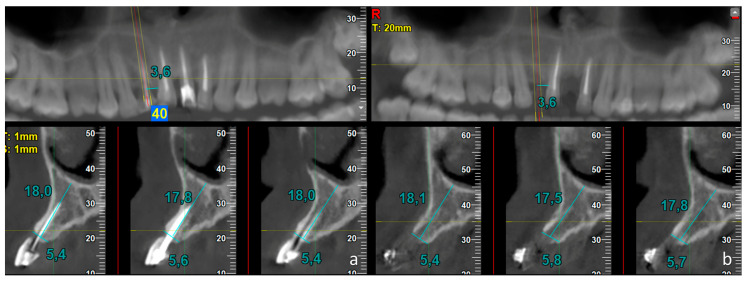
Test site. Cone-beam computed tomographic (CBCT) scans obtained (a) before and (b) 5 months after tooth extraction. In the panoramic view, the central position of the extracted root was identified. At T1, measurements of crestal width and vertical distance from the nasal floor (blue lines) were performed on the central cross-section (red line) and on the adjacent sections located 1 mm mesially and distally (yellow lines). At T2, the distance from the neighboring tooth was determined on the panoramic view, and the same measurements as at T1 were repeated (horizontal blue lines).
